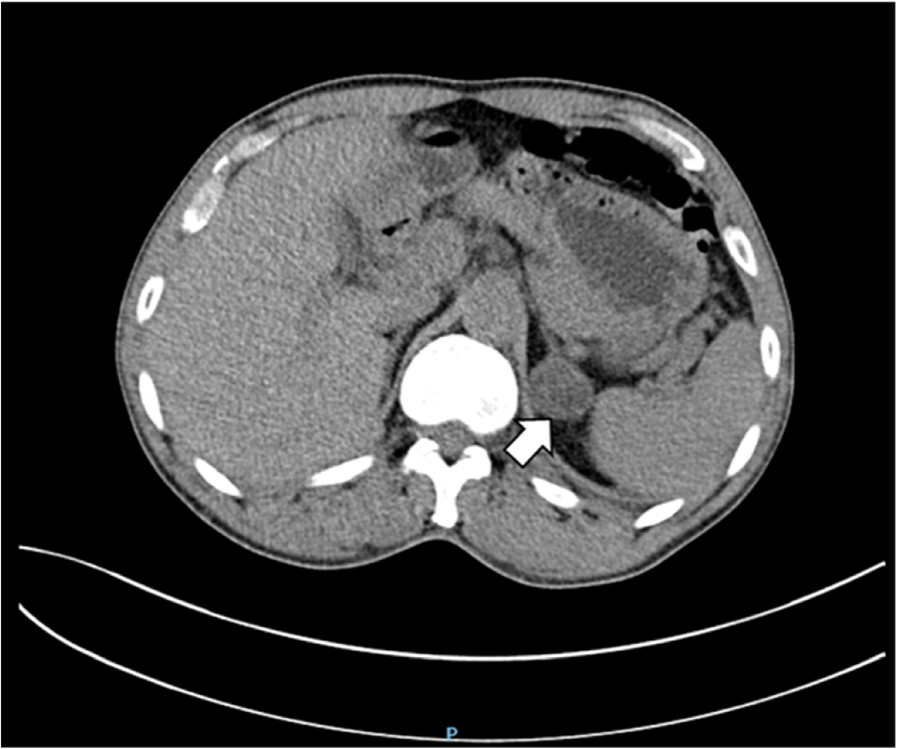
Plain computed tomography scan shows mass in the left adrenal gland. Arrow: left adrenal tumor
